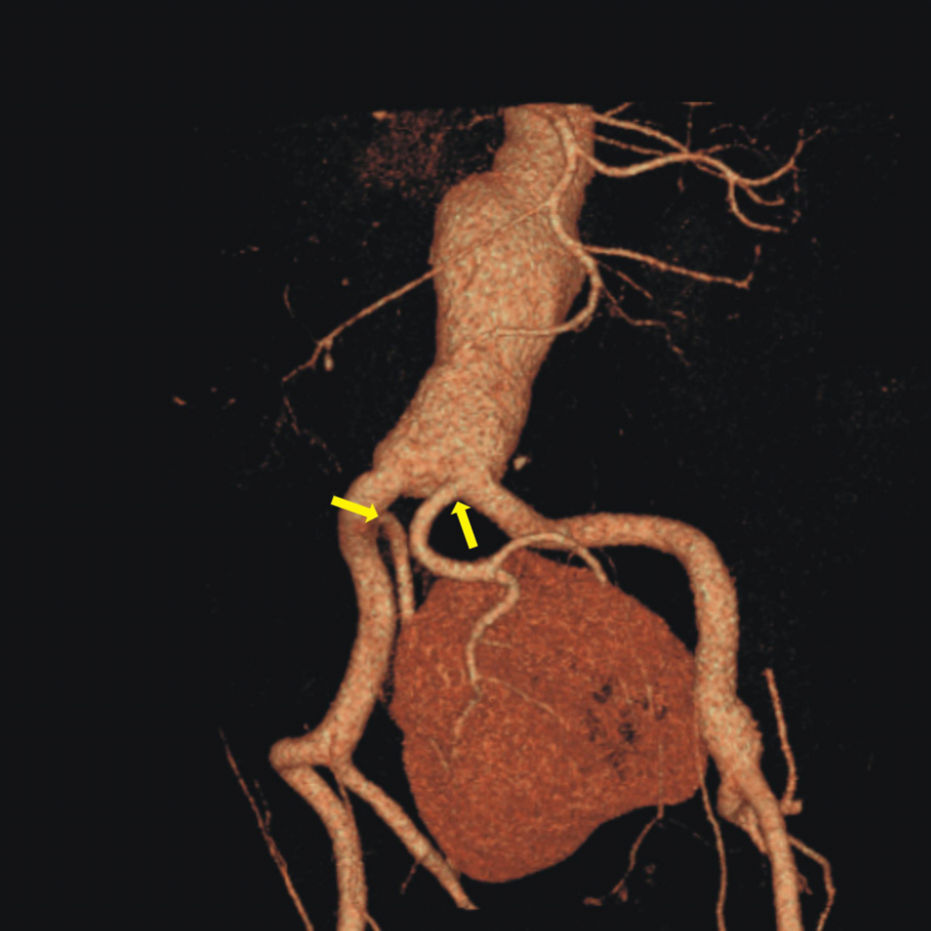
Pre-operative computed tomography angiography of a 59-year-old patient revealed an asymptomatic abdominal aortic aneurysm, and a solitary pelvic kidney, and the composition of the renal vasculature. One renal artery originated from the aortic bifurcation and the other from the proximal portion of the right common iliac artery (yellow arrows).
